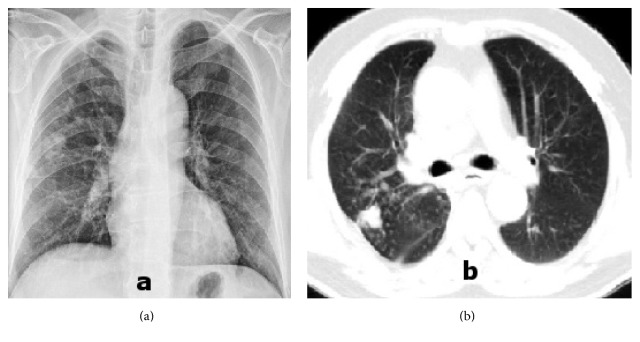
Pretreatment chest X-ray revealed a pulmonary nodule (a) and CT scan showed a nodule in the right upper lobe with tree-in-bud appearance in the adjacent parenchyma (b).
